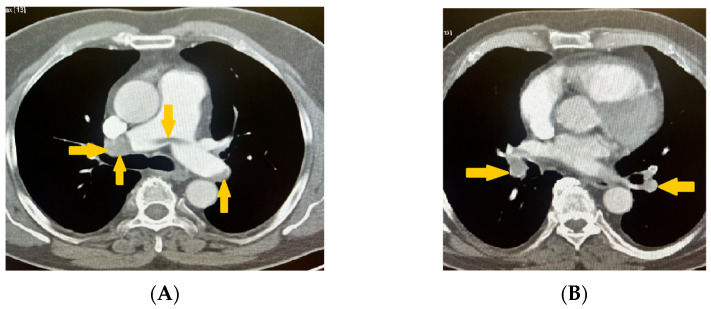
(A,B) CT pulmonary angiography with 3D reconstruction imaging the right and left pulmonary arteries exhibiting an extensive saddle-shaped filling defect with intraluminal occlusions bilaterally, including the interlobar and segmental branches (yellow arrows).
